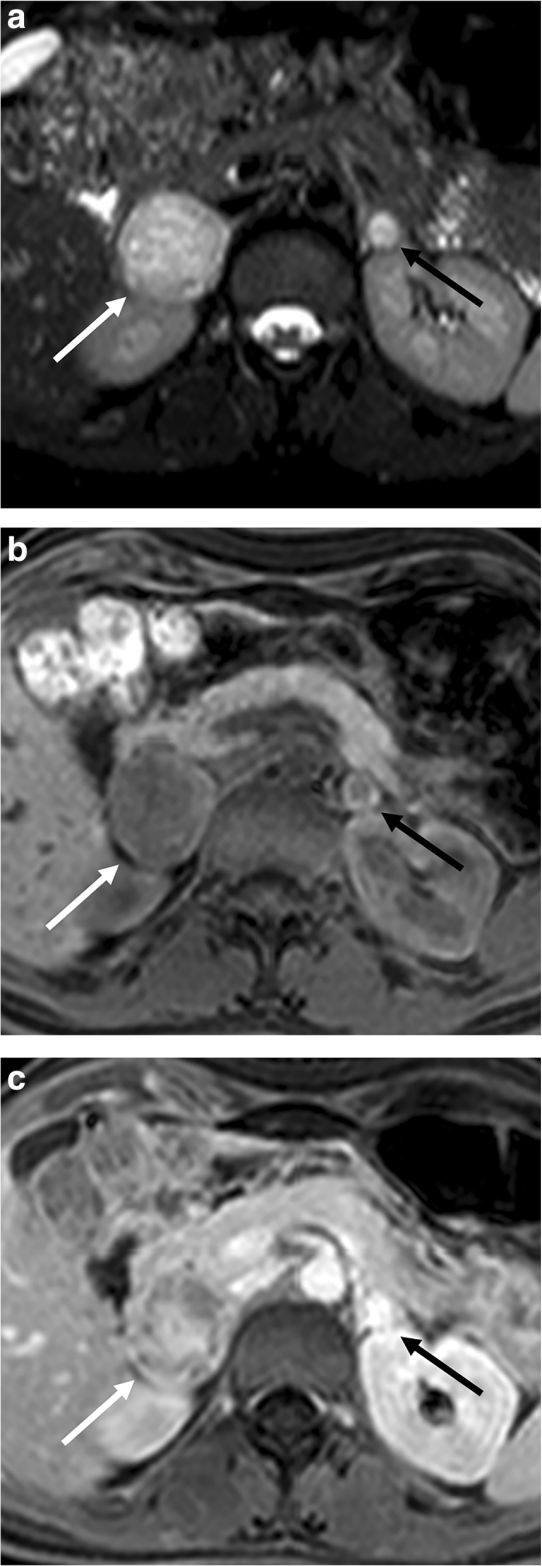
Bilateral adrenal pheochromocytoma in a 27-year-old woman with multiple endocrine neoplasia type 2. Axial T2-weighted fat-saturated spin echo (a), three-dimensional fat-suppressed T1-weighted gradient recalled echo images before contrast injection (b), and in portal phase (c) images show a bilateral adrenal mass with high T2 signal intensity and strong and heterogenous contrast enhancement. The signal intensity is typically more inhomogenous in larger lesions (white arrow, right adrenal gland) than in smaller ones (black arrow, left adrenal gland)
